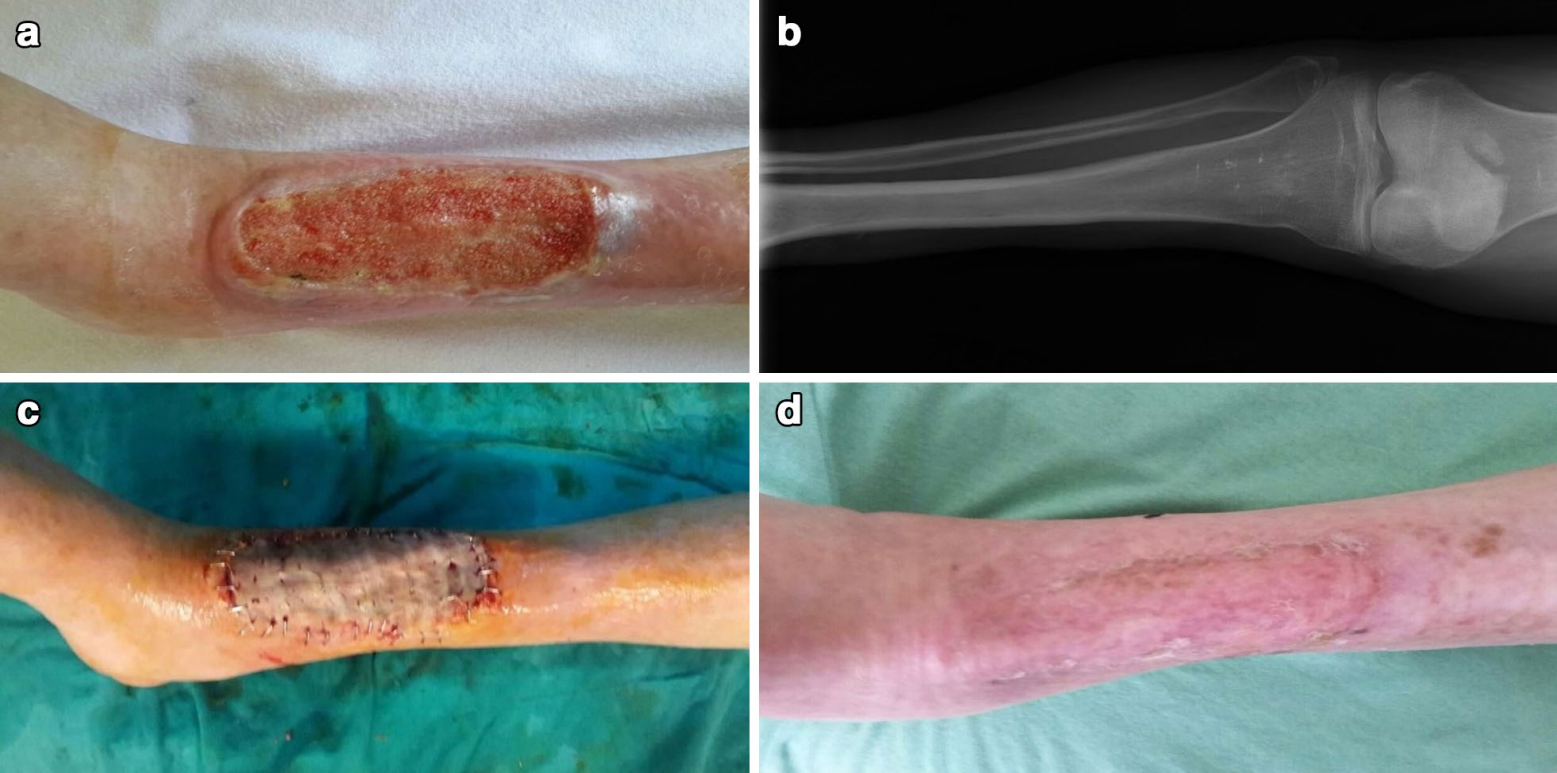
a Preoperative view of the case. b Preoperative X-ray of the left leg with osteopenia. c Early postoperative view of the case. d Late postoperative view of the case after 6 month
